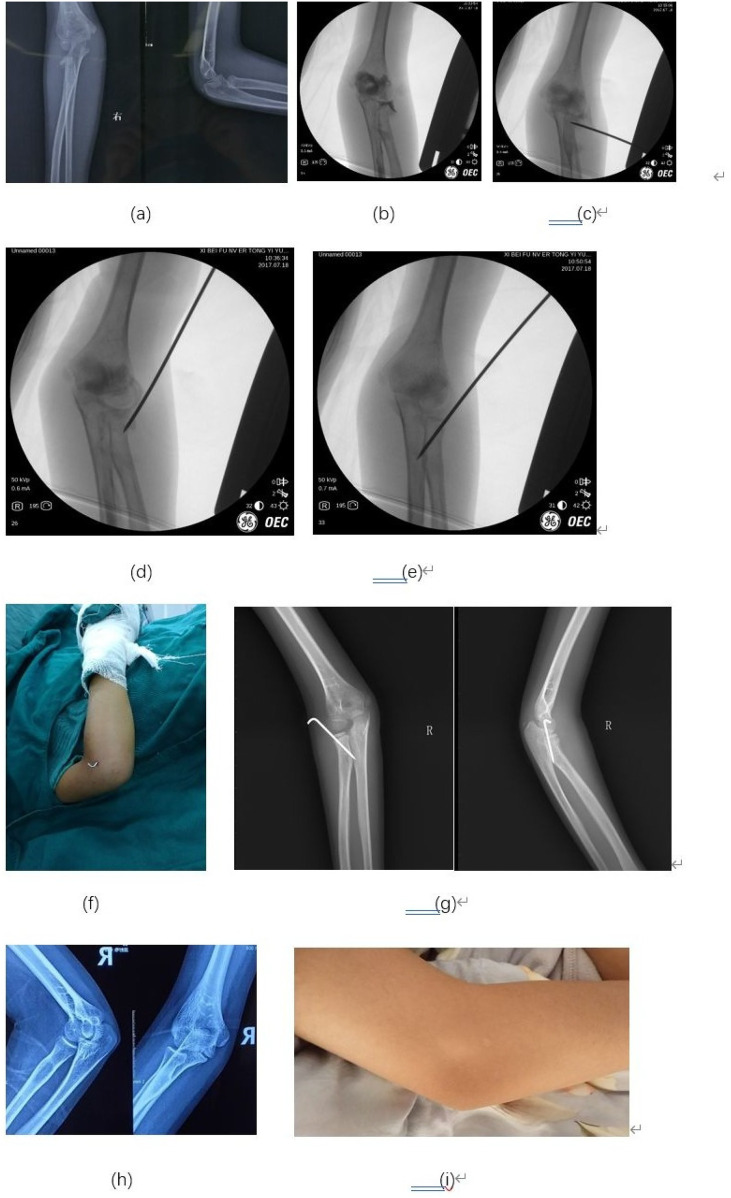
Typical case: An 8-year-old girl presented with a right elbow injury. (a) The preoperative x-ray showed 62° angulation of the radial neck fracture. (b) Arthrography provided clearer visualization of the radial neck angulation, more accurately delineating the fracture extent. (c) A 2.0 mm K-wire was inserted laterally and positioned distal to the fracture site. (d) Leverage reduction was performed using the K-wire to realign the fracture fragments. (e) The K-wire was further advanced to penetrate the cortex, providing additional stability to the reduced fracture. (f) The external portion of the K-wire was carefully removed, ensuring secure fixation while minimizing soft tissue irritation. (g) Anteroposterior (AP) and lateral projection (LP) x-rays taken one month postoperatively demonstrated successful union of the fracture. (h) AP and lateral x-rays at 7.5 years demonstrated excellent humeroradial and proximal radioulnar congruity, confirming long-term stability. (i) At 7.5 years, only a faint scar remains on the lateral elbow, yielding minimal cosmetic impact.
